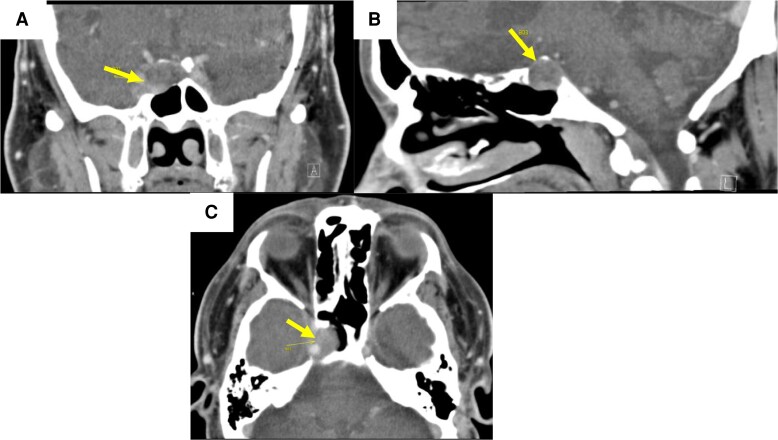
Computed tomography scan of the brain from case 1. Arrows are pointing to the mass in all images. A, Coronal section of the sellar mass medial to the right cavernous sinus. B, Sagittal scan showing the mass extending into the sphenoid sinus. C, Axial section showing mild contrast enhancement of the mass abutting the superior orbital fissure.
